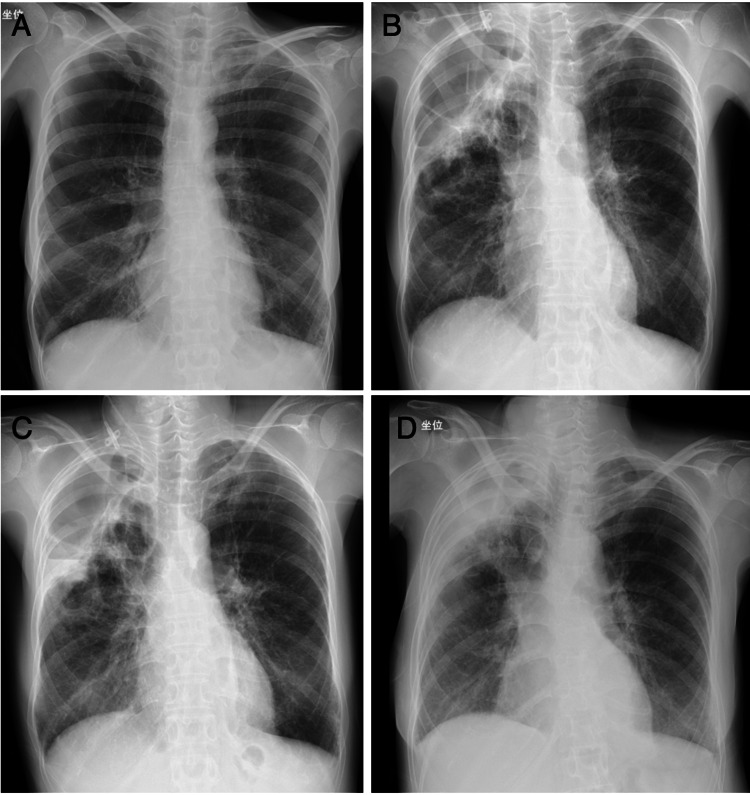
Chest x-ray images of the patient. Chest x-ray images (A) one year earlier, (B) on admission to Hamanomachi Hospital, (C) before percutaneous drainage on day 38, and (D) before discharge from Hamanomachi Hospital on day 87.
